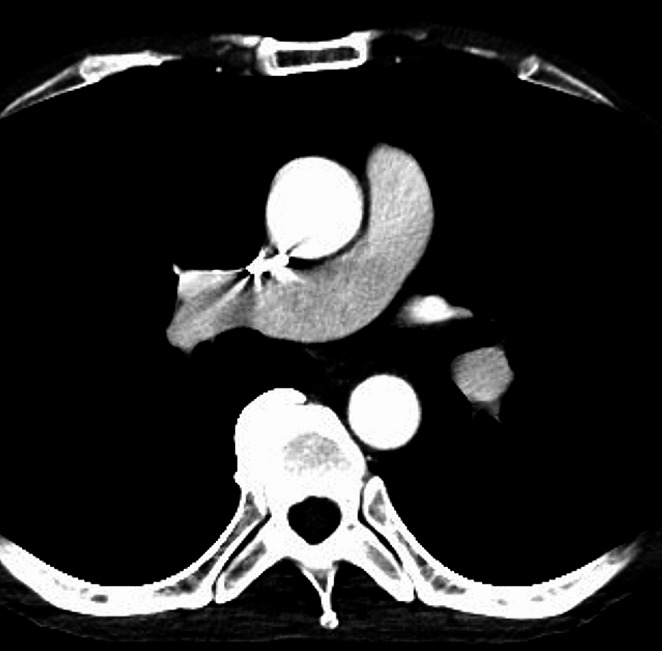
CT pulmonary angiography of a 48-year-old male in whom transient interruption of contrast was seen. The contrast material was injected from right upper extremity in this patient. The CT attenuation of pulmonary artery and descending aorta was 144 and 277 Hounsfield unit, respectively. While high-attenuation contrast material can be seen in both the superior vena cava and aorta, CT attenuation of pulmonary artery is relatively low. This phenomenon would be possibly due to mixture of blood from left upper extremity and inferior vena cava, whose CT attenuation is low, with the inspiration maneuver during scan
